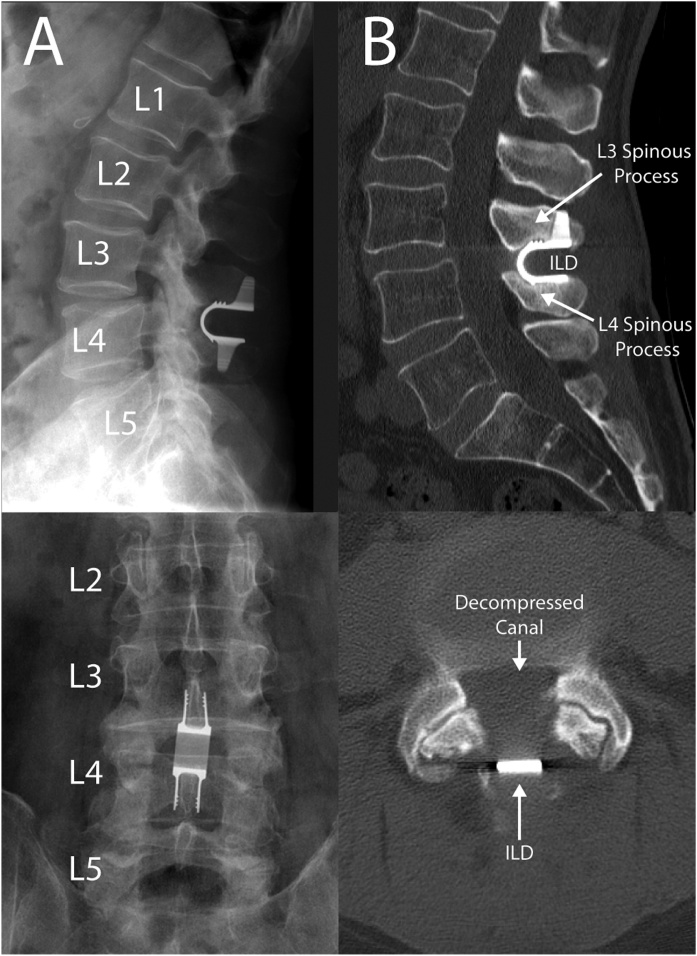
A) Post-operative X-Rays (lateral-top, antero-posterior-bottom) showing ILD at L3-4 obtained on post-operative day 1. B) 6-month post-operative CT (sagittal-top, axial-bottom) shows hardware in place with no evidence of cyst recurrence.
